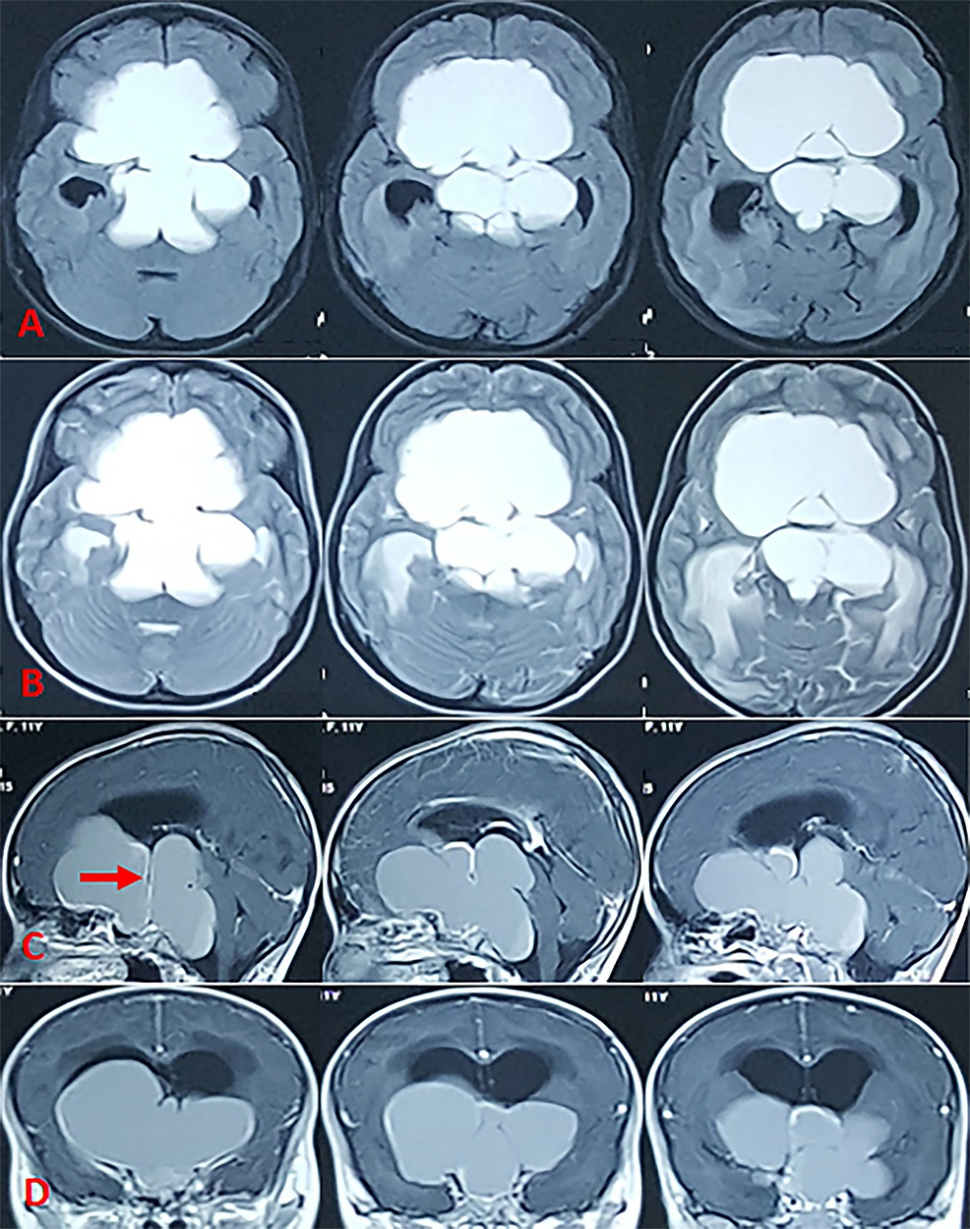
MRI of the brain Brain MRI showing a diffuse hyperintense signal on axial fluid-attenuated inversion recovery (FLAIR) (A) and axial T2-weighted images (B), which is consistent with high levels of protein and cholesterol. Sagittal (C) and coronal (D) sections of postcontrast T1-weighted images reveal no significant enhancement of the solid component. All these findings support the diagnosis of cystic craniopharyngioma. The lesion extended from anterior to posterior fossa and laterally to the middle fossa, compressing and elevating all basal elements (frontal lobes, temporal lobes, third ventricle, brain stem). The chronically compressed pituitary infundibulum has been elongated and pushed superiorly as shown (arrow in C).
